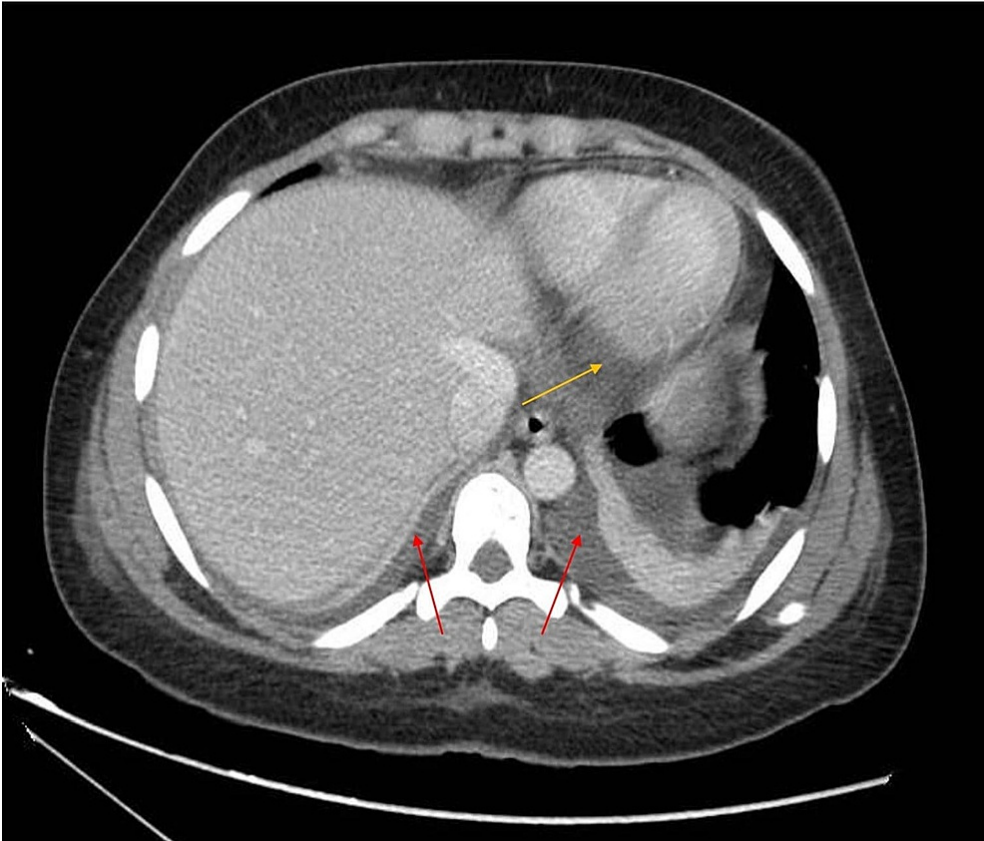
CT chest showing pericardial (yellow arrow) and bilateral pleural effusion (red arrows)
